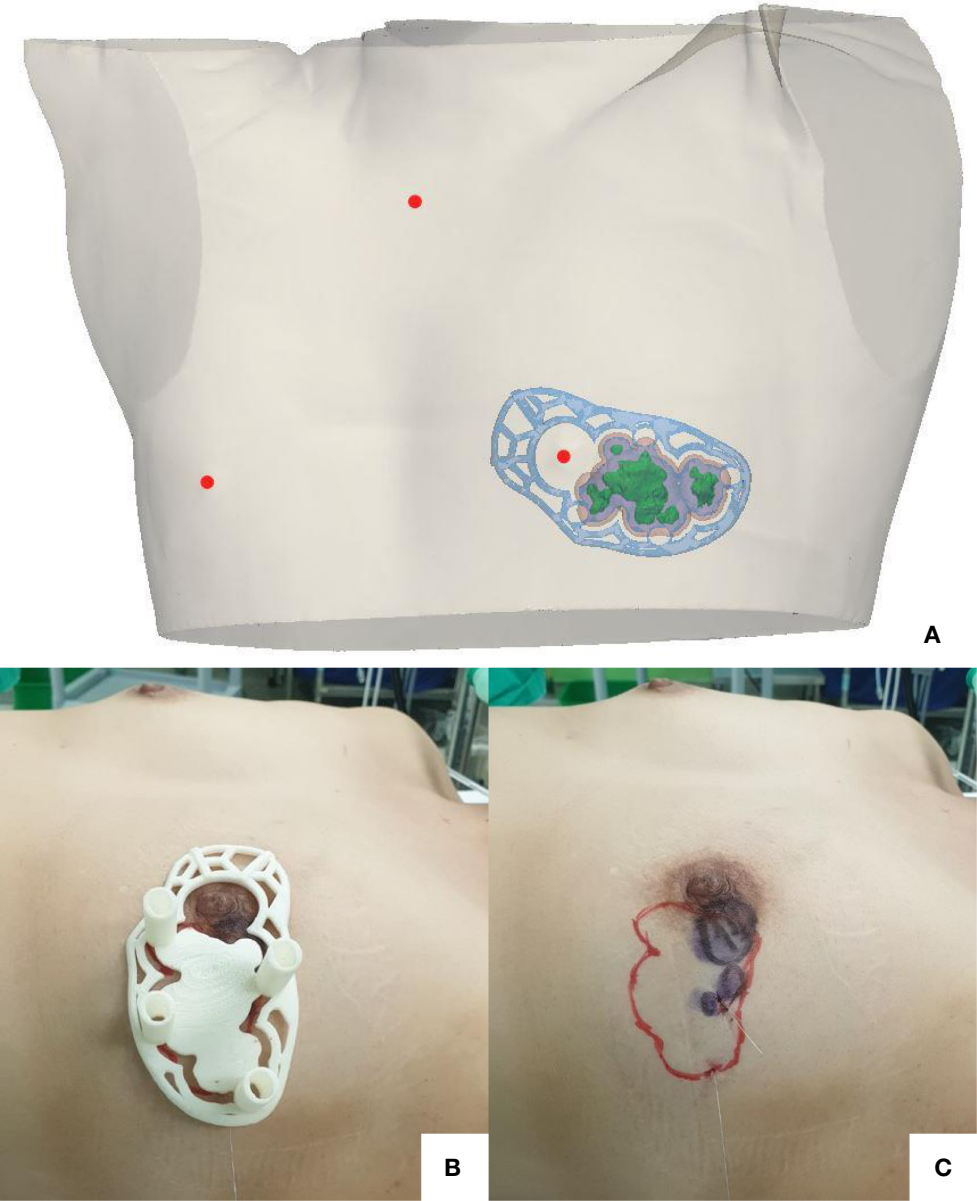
The breast surgical guide (BSG) targeted the original tumor region by combining pretreatment prone magnetic resonance imaging (MRI)-generated tumor data and posttreatment supine MRI-generated breast data (A). The BSG was manufactured as a hybrid type, containing a groove for marking the original tumor area on the breast surface with an extra 0.5 cm from the tumor boundary to guarantee safe margins. Blue dye injection columns were provided to indicate the extent of the tumor requiring removal (B). A surgical resection line was drawn onto the breast skin surface along with a groove designed to match the original tumor area (C).
